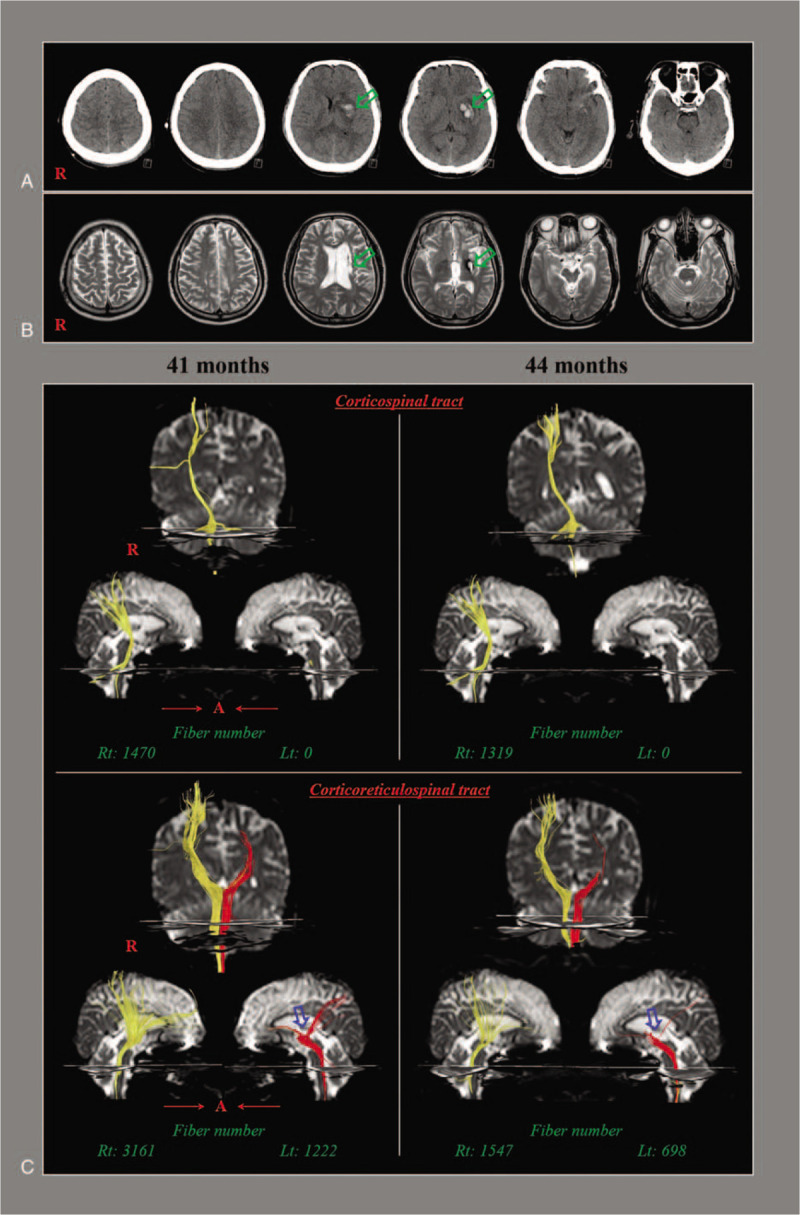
(A) Brain computed tomography at onset show traumatic intracerebral hemorrhage (arrows) in the left basal ganglia and subarchnoid hemorrhage. (B) T2-weighted brain MR images at 41 months after onset reveal leukomalactic lesions (arrows) in the left corona radiata and basal ganglia. (C) Diffusion tensor tractography (DTT) for the corticospinal tract (CST) and the corticoreticulospinal tract (CRT). The fiber number of the right CST is decreased on 44-month DTT (1319) compared with 41-month DTT (1470) and the left CST is not reconstructed on both DTTs. The fiber number of both CRTs are decreased on 44-month DTT (right: 1547, left: 698) than 41-month DTT (right: 3161, left: 1222). The left CRT shows partial tearing in the subcortical white matter (arrows) on both DTTs.
